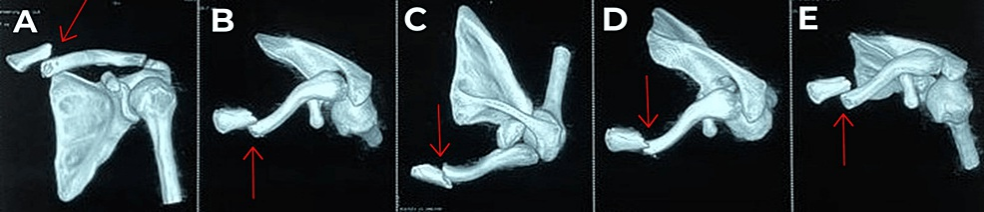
3D CT showing extra-articular displaced medial end clavicle fracture Figures A, B, C, D, and E show multiplanar 3D views of the fracture Red arrows indicating the fracture site 3D CT: three-dimensional computed tomography
